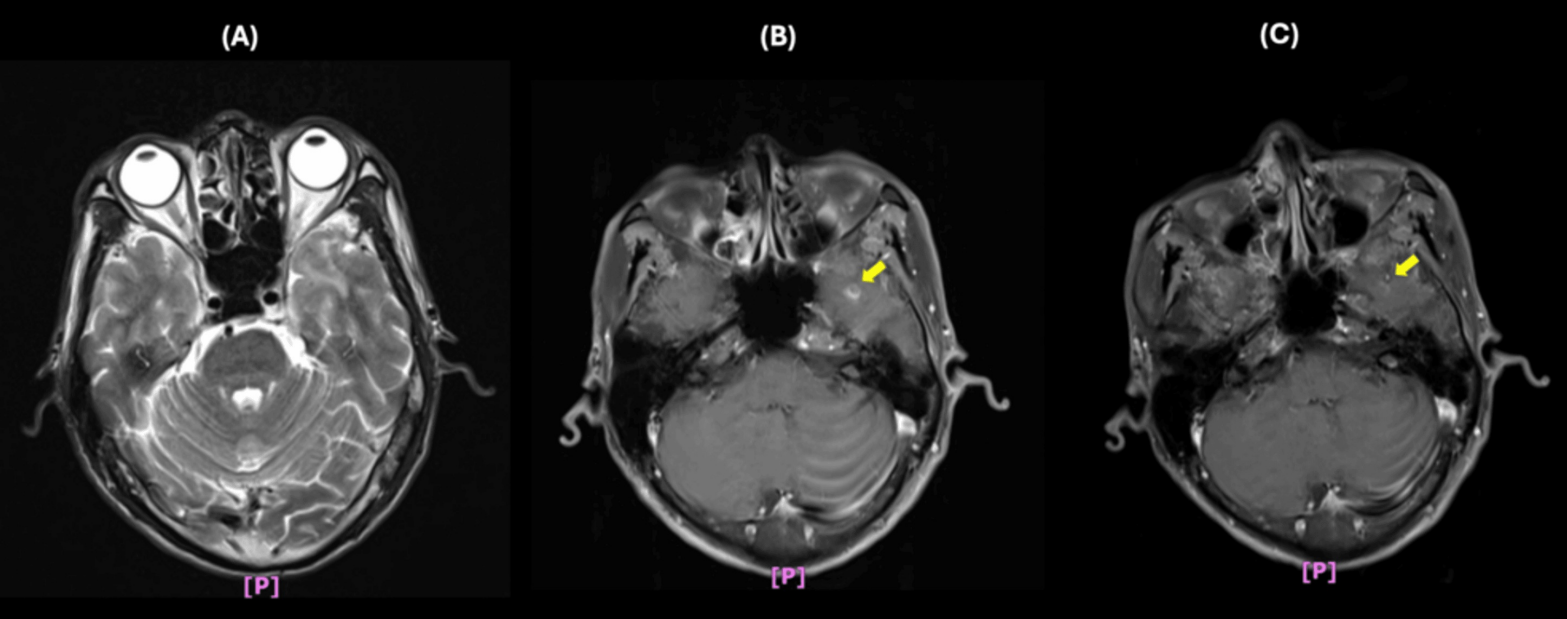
MRI of the nasopharynx (A) May 2017 MRI showing mild white matter changes on T2-weighted imaging. (B) May 2017 MRI revealing an enhancing nodule on the T1 turbo spin echo fat-suppressed sequence (arrow). (C) August 2017 follow-up MRI demonstrating regression of the enhancing nodule. MRI: magnetic resonance imaging
